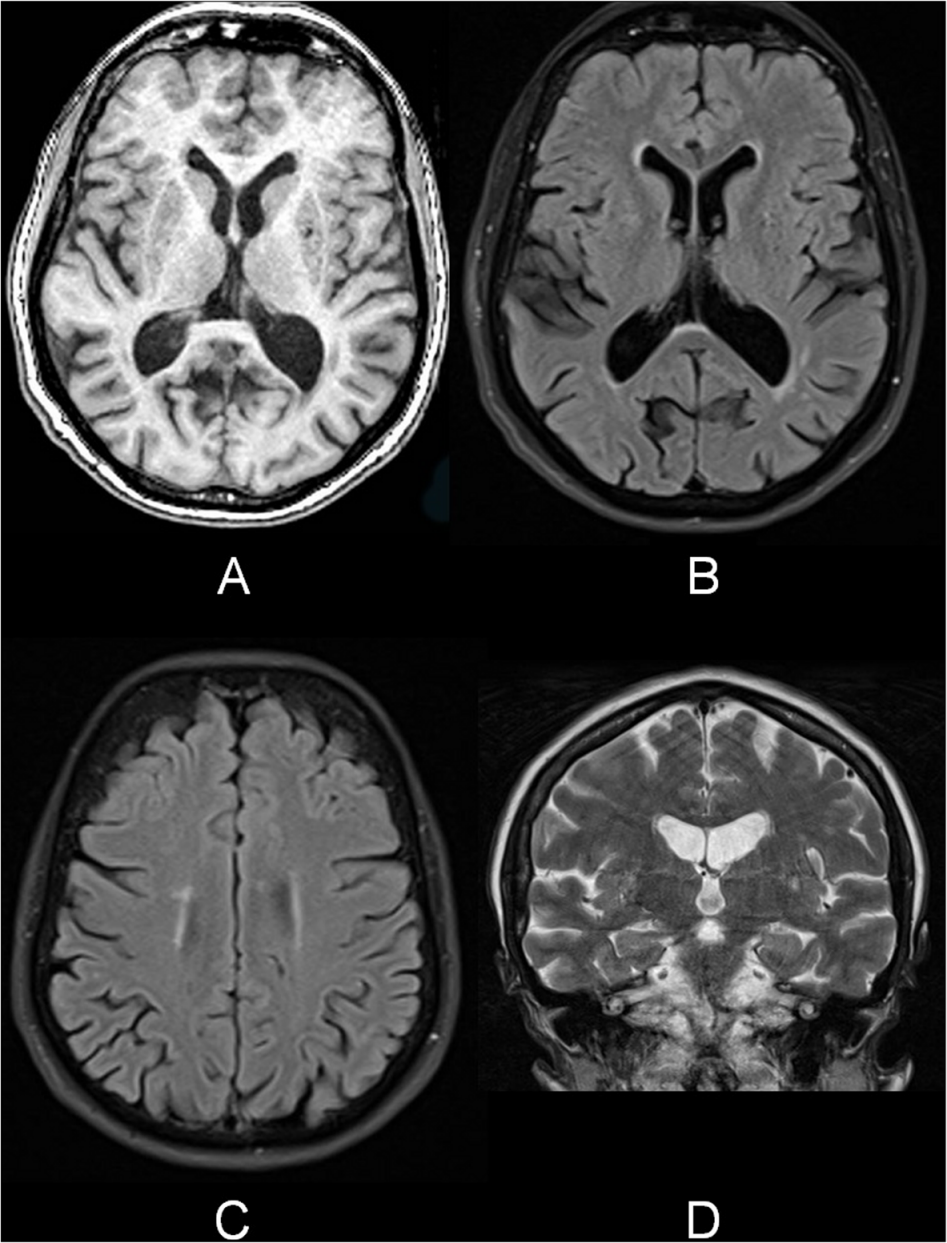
Magnetic resonance imaging scan of the brain. A, transverse T1-weighted image; B and C, transverse T2-weighted fluid attenuated inversion recovery image; D, coronal T2-weighted image of the medial temporal lobe and hippocampus. There was global cortical atrophy grade 1-2 with mild atrophy of the hippocampus (medial temporal lobe atrophy score, 1-2), on the left more pronounced than on the right. Compared to a magnetic resonance imaging scan performed one year earlier, there was no progression of these features. Furthermore, the T2-weighted images showed some punctuate subcortical hyperintensities but no other focal structural changes.
